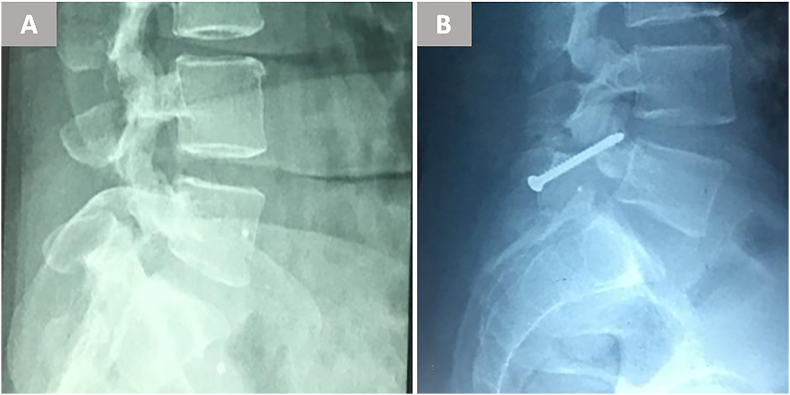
Lateral radiographs of a 28-year-old male with incomplete pars fusion at 18-month follow-up. Despite lack of complete fusion, the patient reported clinically meaningful improvement (fair outcome), potentially due to fibrous stabilization reducing micromotion.
